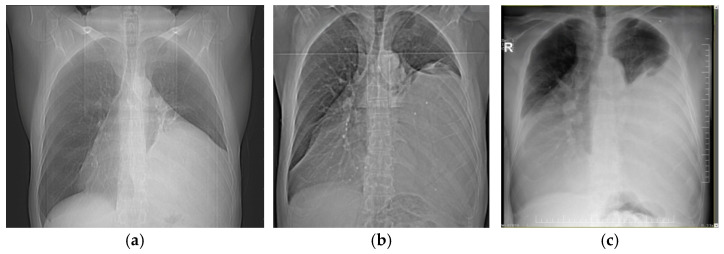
Conventional chest radiography of the patient from the presentations in 2008 (a), 2018 (b), and 2023 (c) showing significant growth over time of the dense opacity caused by the tumor, with massive compression of both lungs and mediastinal shift towards right hemithorax.
